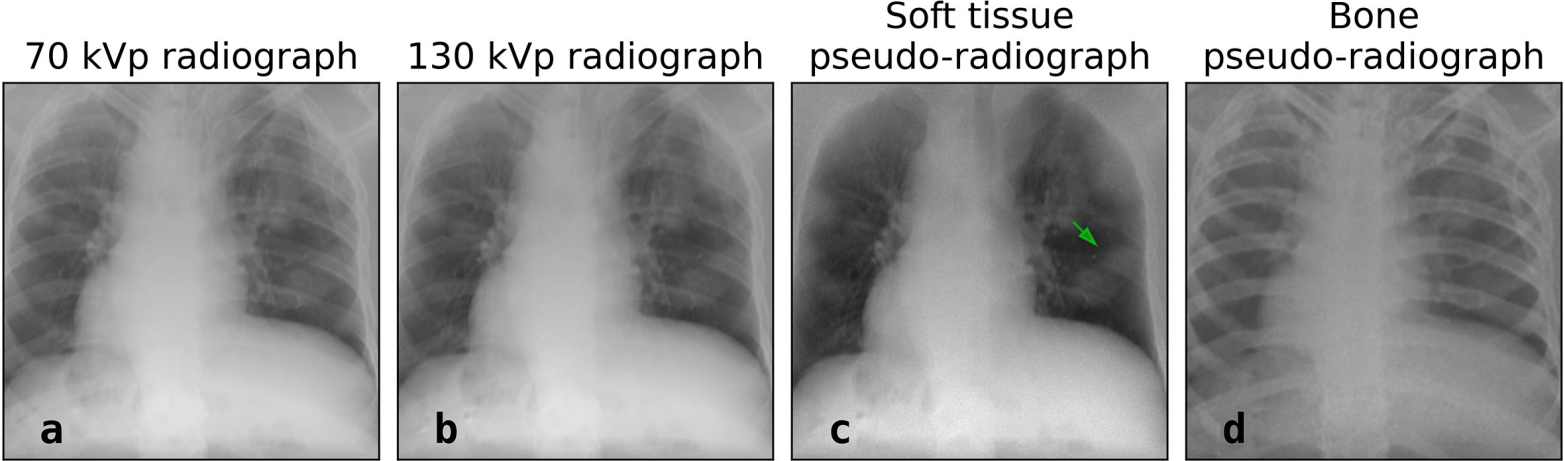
The low (a) and high (b) energy X-ray radiographs, and the post-processed pseudo-radiographs representing the soft-tissue (c) and the bone content (d) in the chest of a patient diagnosed with COVID-19 pneumonia. (Data set: coronacases_002.nii.gz from Jun et al^12^)
